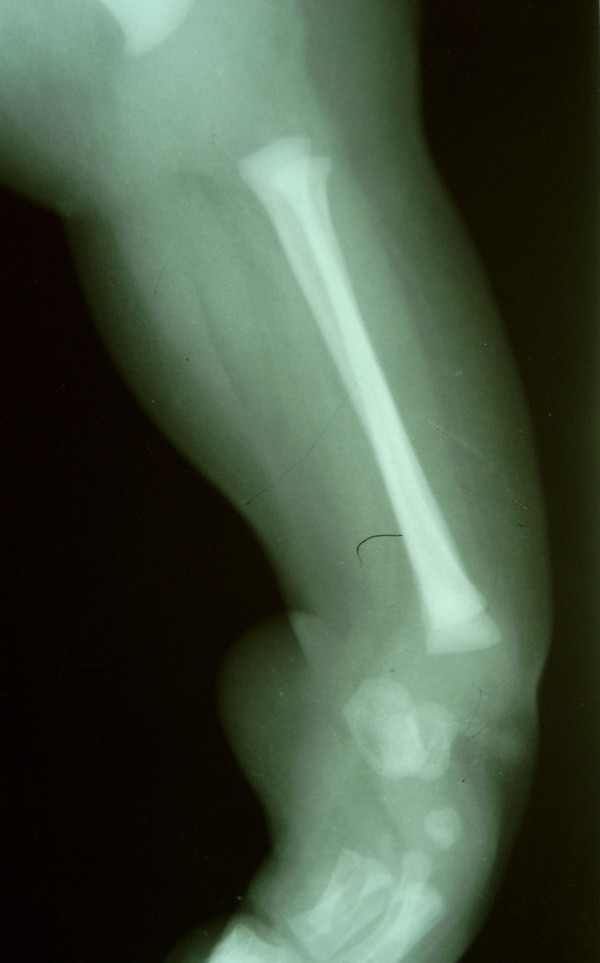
Lateral radiograph of the left leg showing anterior subluxation of the distal end of the tibia over the talus.
